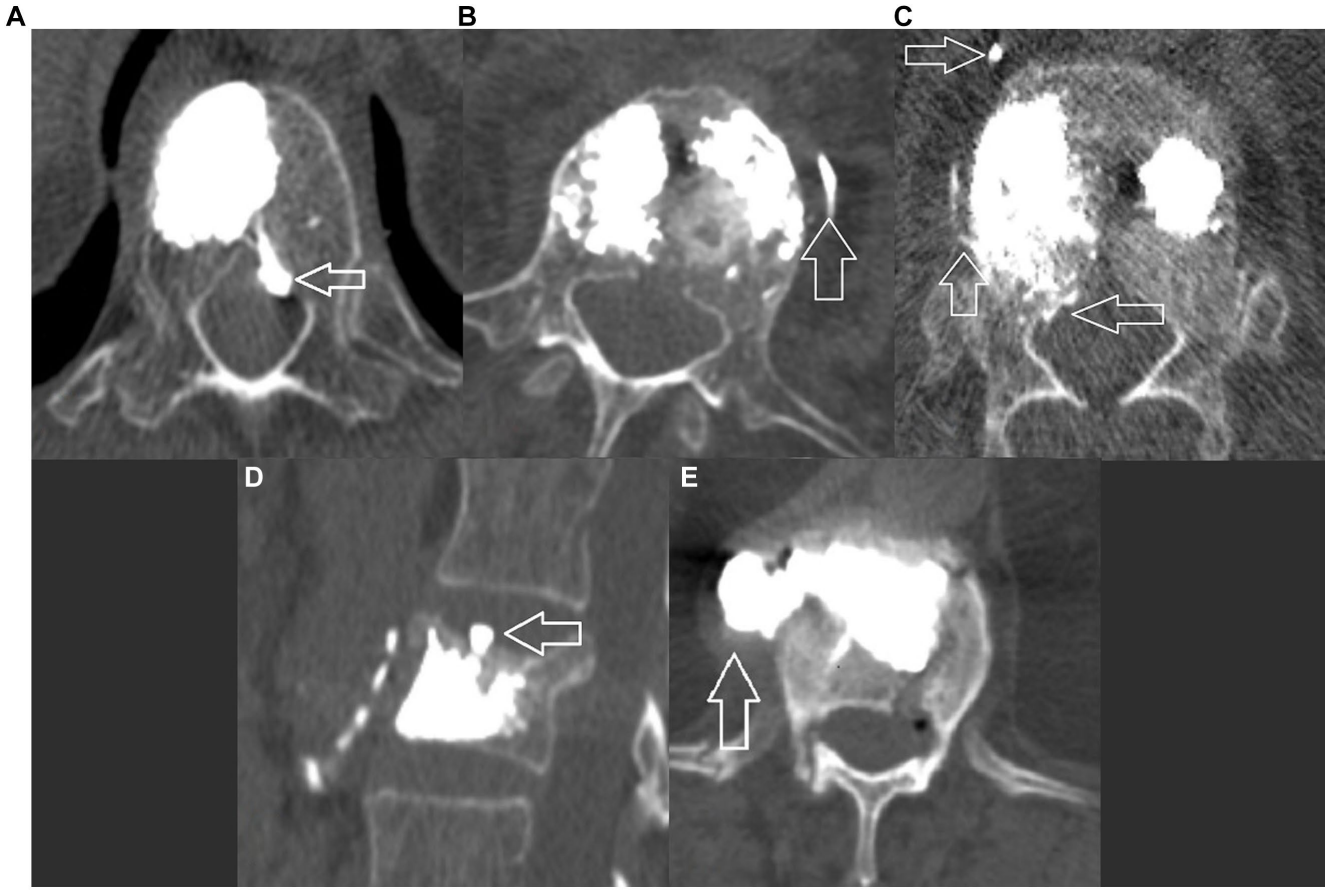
Postoperative CT, axial view showing different categories of leakage: (A) Type A – inside the spinal canal through the basivertebral vein; (B) Type B – inside the segmental veinous outflow; (C) Type C – inside the spinal canal and the segmental veinous outflow; (D) Type D inside the intervertebral disk; and (E) Type E – in the paravertebral space, leak occurs through a cortical defect.
